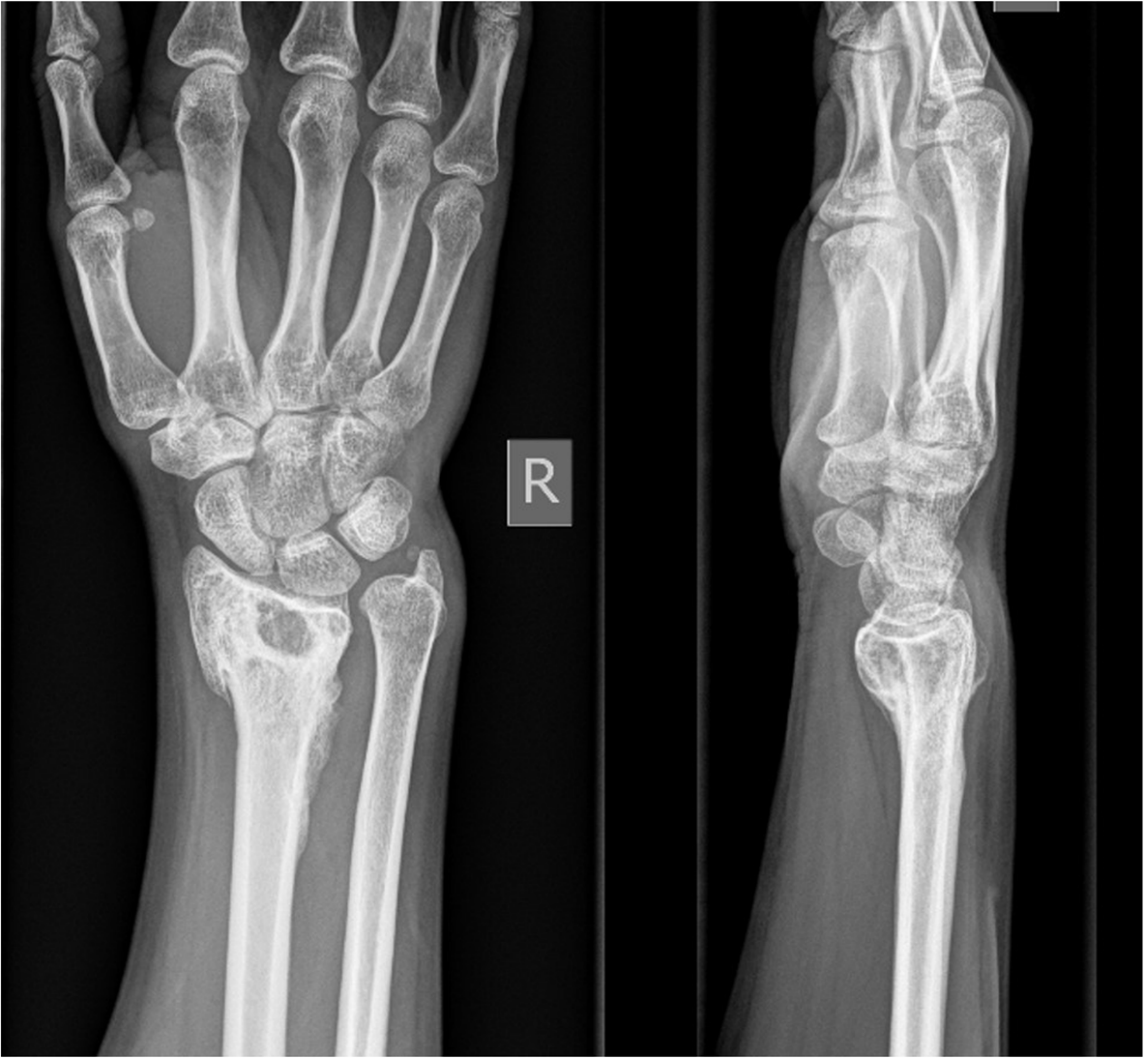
ap and lateral plain radiographs of right wrist 4 years after treatment
